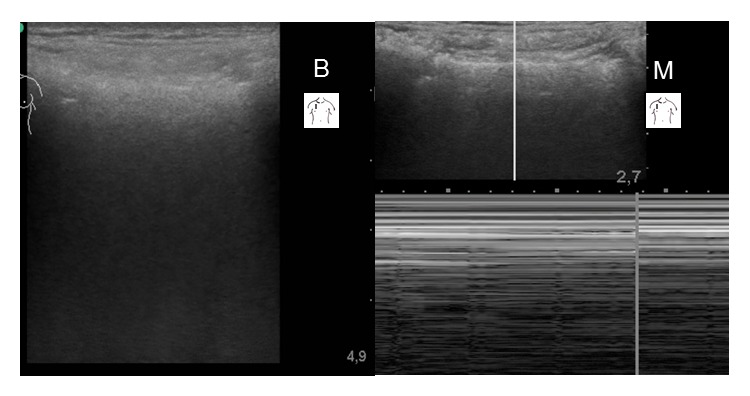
Case 2: B-mode and M-mode sonography using a linear transducer positioned in the mid-clavicular line at the 3rd right intercostal space (B: B-mode, M: M-mode).
